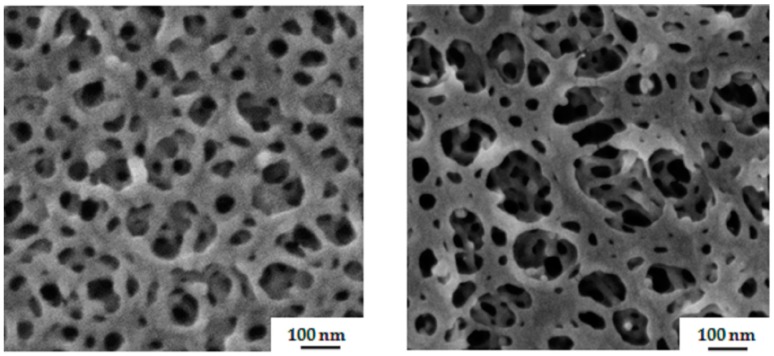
SEM topography images of the surface of the generated membrane from 25 wt % PS_76_-PSMA_24_^200^ in THF/DMF 30/70 wt % with evaporation time 10 s (left) and 20 s (right).
